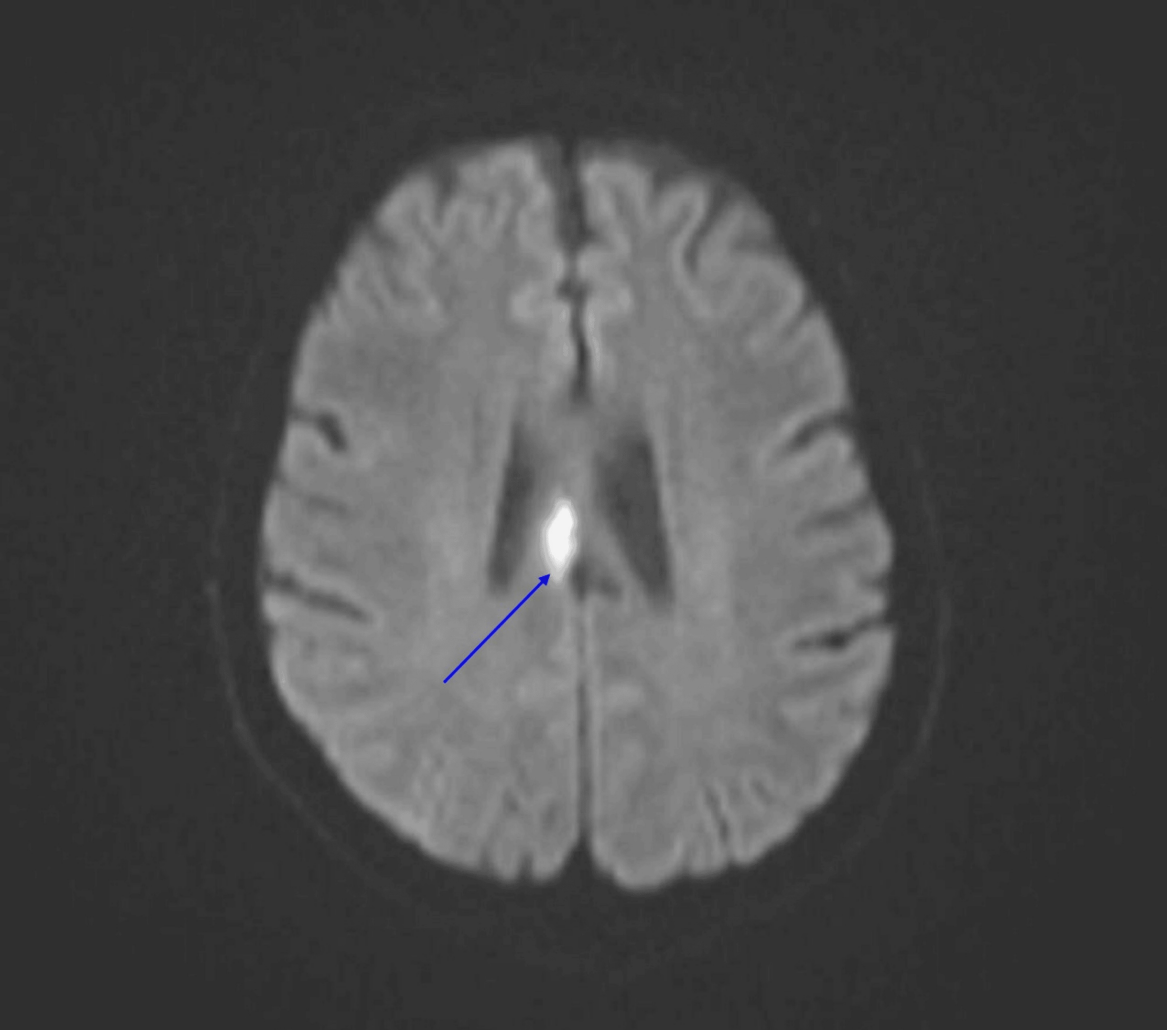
MRI brain, axial view, DWI sequence, showing diffusion restriction in the right body of the corpus callosum. DWI: diffusion-weighted imaging.
